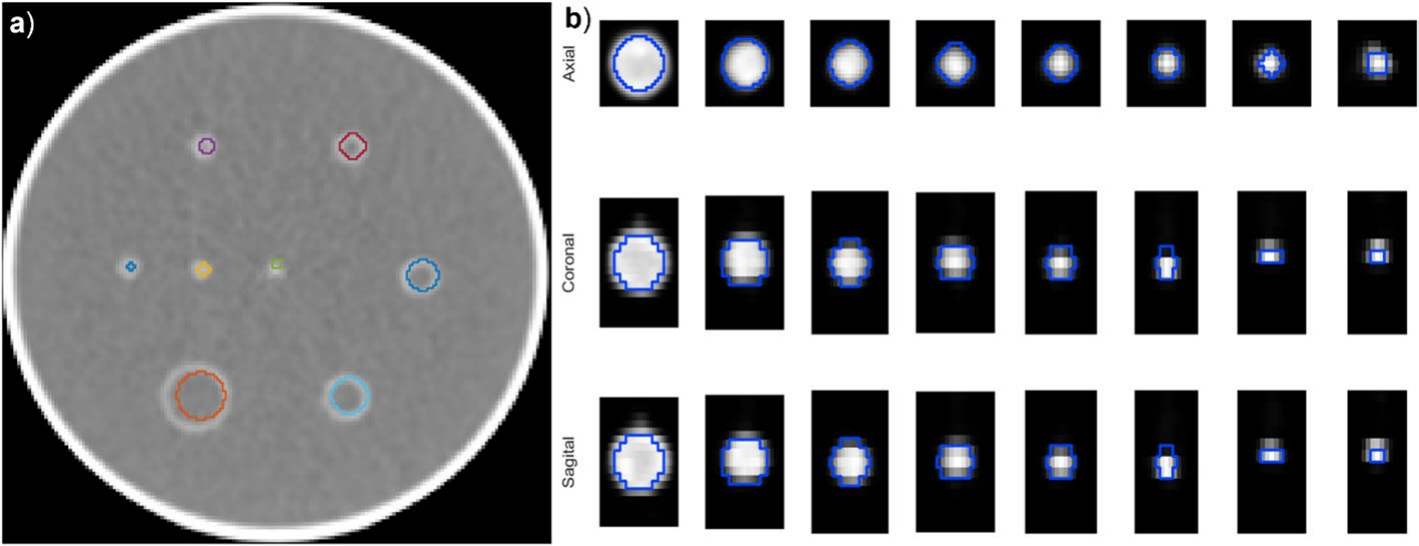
Example delineations of VOIs for each sphere shown on the CT (a) and PET image (b) in all three directions
